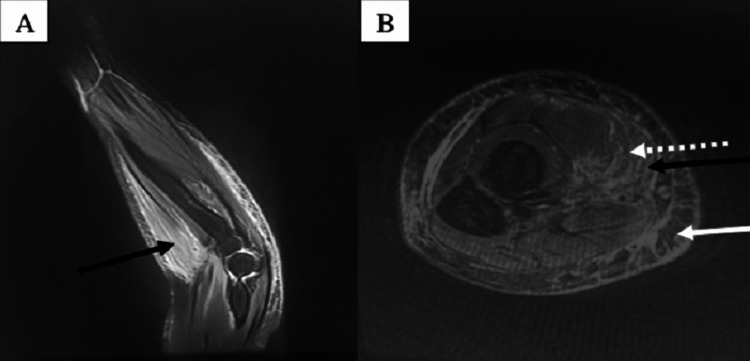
The MRI of the left forearm A: Proton density (PD) fat-saturated sequence, sagittal view; B: T1-weighted fat-saturated post-gadolinium injection sequence, axial view, showing myositis (black solid arrow) and fasciitis (white dotted arrow) affecting the anterior compartment of the left forearm and edema of the subcutaneous tissues (white solid arrow)
